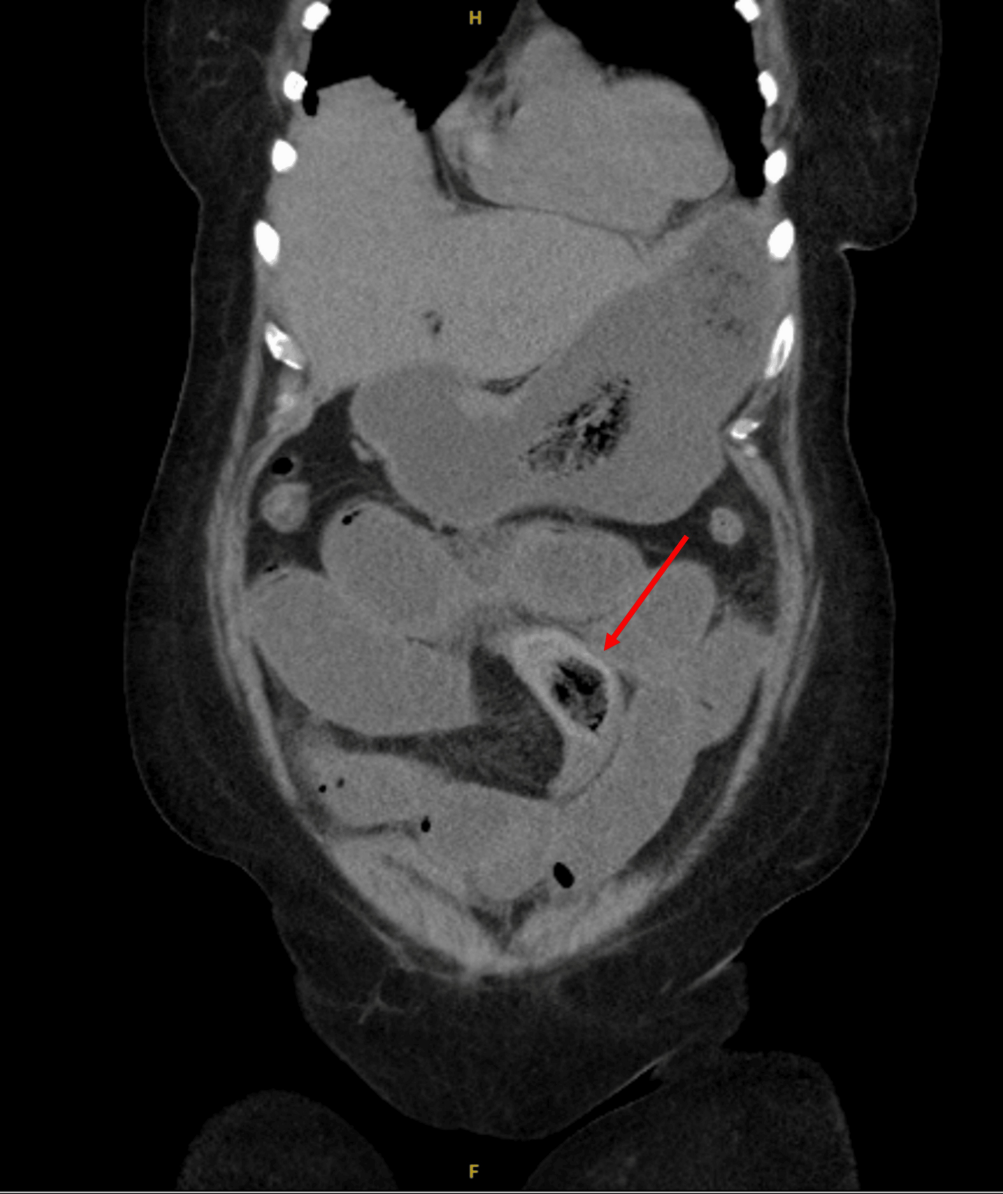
Non-contrast CT scan, coronal view, displaying a small bezoar seen in the mid abdomen (red arrow).
